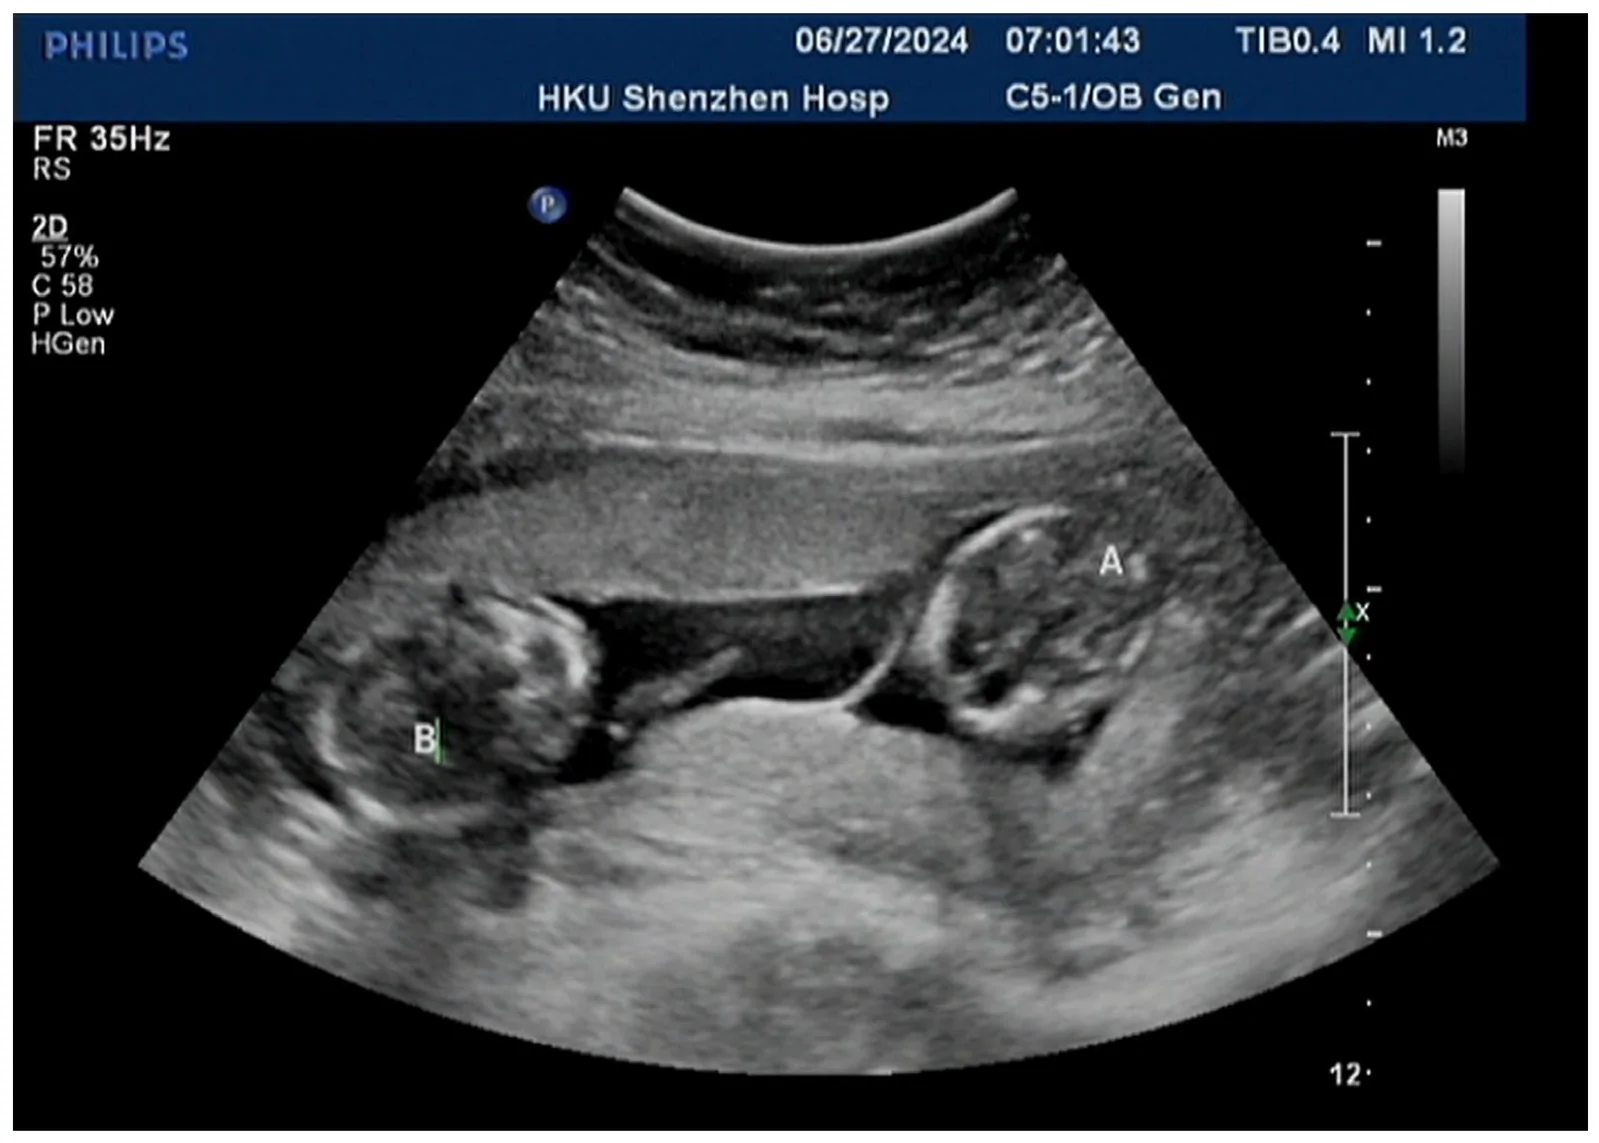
Ultrasonography shows two viable fetuses with normal amniotic fluid volume, indicating a twin pregnancy. “A” and “B” denote twin A and twin B.
